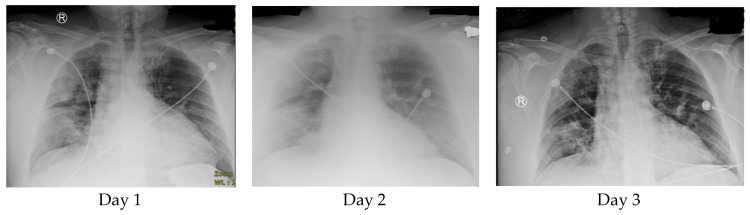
Chest X-rays on day 1, day 2, and day 5. Tocilizumab was administered as two doses of 500 mg Q12 (during the night of day 1 and the morning of day 2).
